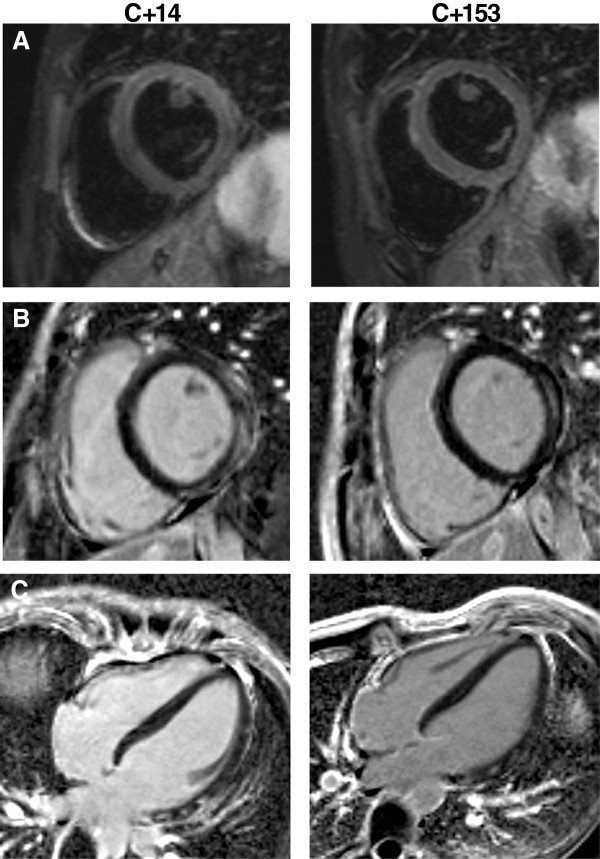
Cardiac MRI on day 14 and 153 after CHMI. (A) Slightly increased T2-weighted signal intensity was observed in the basal-inferolateral segment of the left ventricle on day 14 after CHMI (C + 14), which had disappeared on day 153 after CHMI (C + 153); visualized on the short-axis dark blood STIR (short inversion time inversion recovery) recordings. (B and C) After administration of 15 mL gadolinium contrast subepicardial and midwall delayed enhancement was observed in the basal-inferolateral and basal-inferior segments of the left ventricle on day C + 14, which had decreased on day C + 153; visualized on the short-axis (B) and the 4-chamber (C) PSIR (phase sensitive inversion recovery) recordings.
